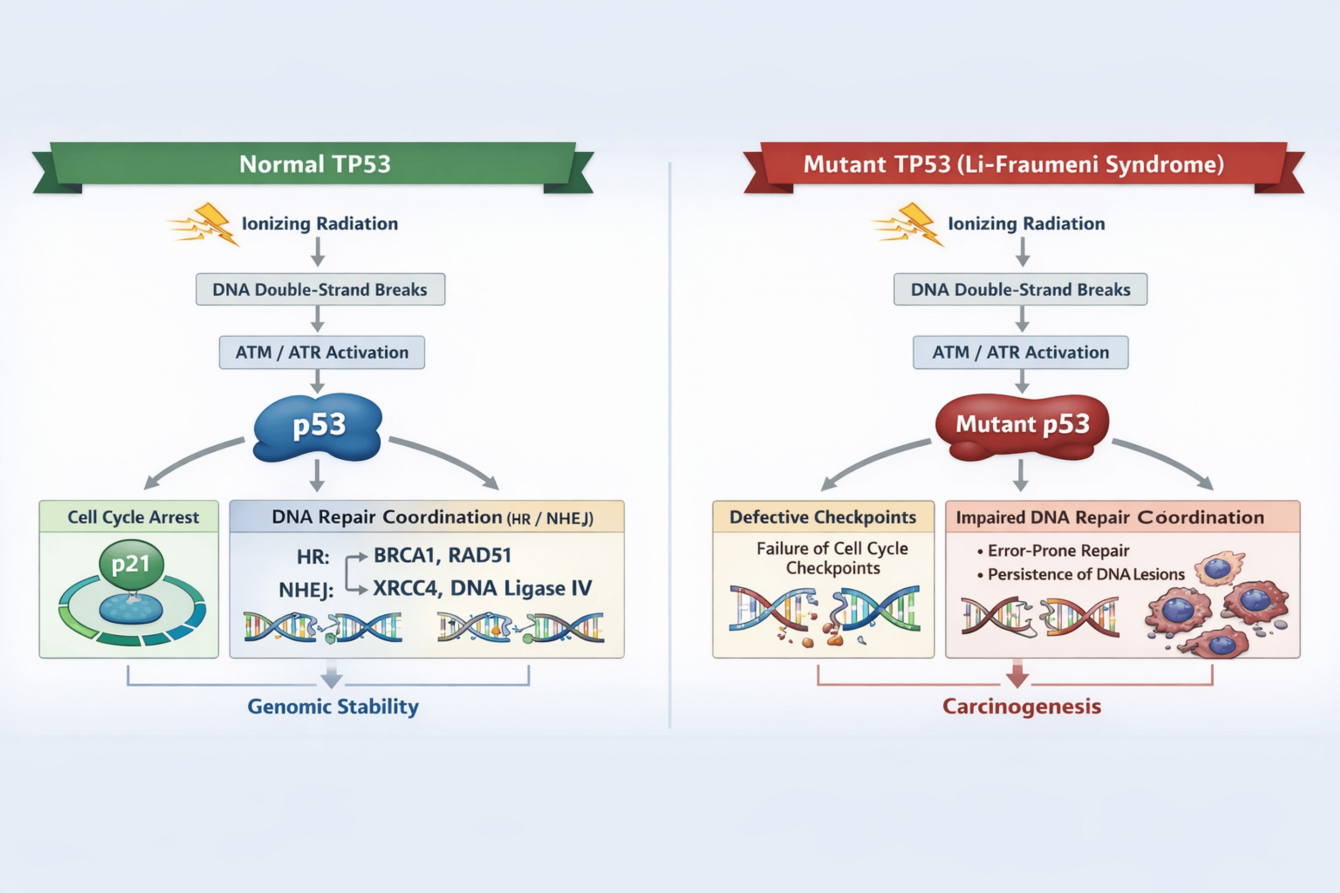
TP53-mediated cellular response to radiation-induced DNA damage in normal cells and Li-Fraumeni syndrome. HR: homologous recombination, ATM: ataxia-telangiectasia mutated, ATR: ataxia telangiectasia and Rad3-related, BRCA1: breast cancer 1, RAD51: RAD51 recombinase, XRCC4: X-ray repair cross-complementing protein 4, NHEJ: non-homologous end joining This figure was created using ChatGPT (OpenAI, San Francisco, California).
